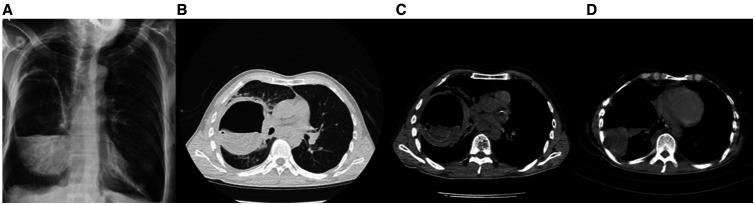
(A) Radiological image showed a cavitary lesion, which is considered a ruptured hydatid cyst. (B–D) Noncontrast chest computed tomography scan showed an air-containing cystic lesion with an internal undulating and collapsed germinative membranes representing detached membranes of hydatid cyst.
